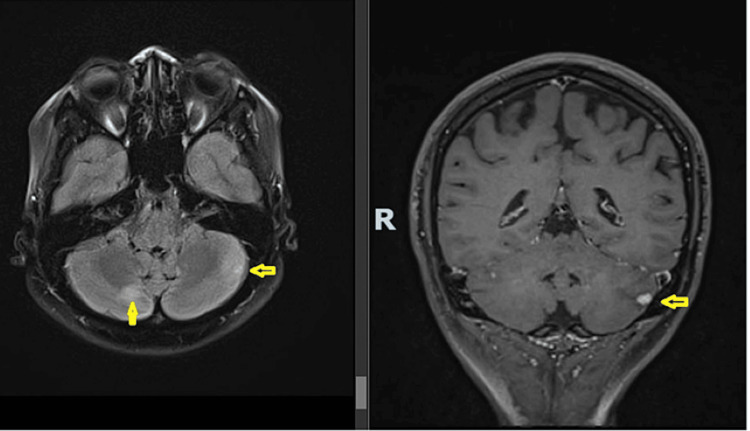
Surveillance MRI of the brain done at 20 weeks gestation, showing stable hemangioma (0.3 cm) in the cerebellar with no new mass and no features of increased intracranial pressure.
